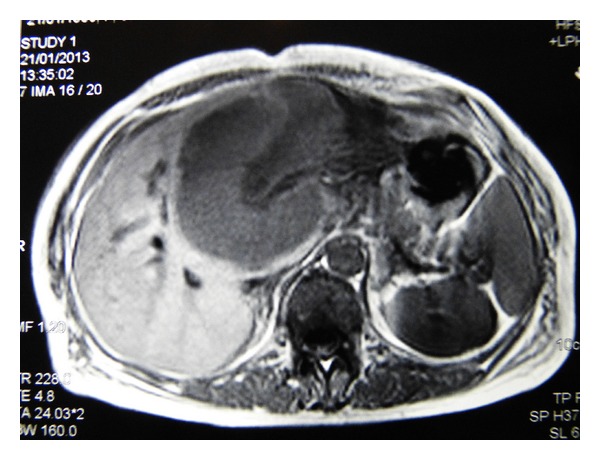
Axial T1-weighted MRI, showing a hypoattenuating hepatic lesion.
